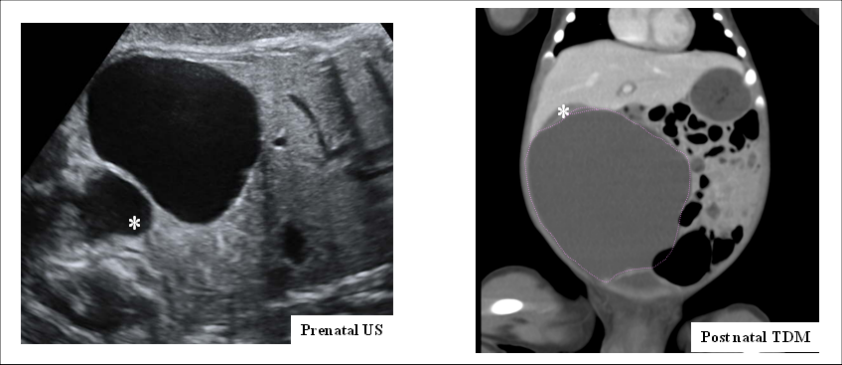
Figure 2: Patient 2 imaging: Prenatal US showing a large intra-hepatic cyst, and a normal gladbladder (*). CT scann at births confirmed a very large hepatic cyst and the normal gladbladder (*).
